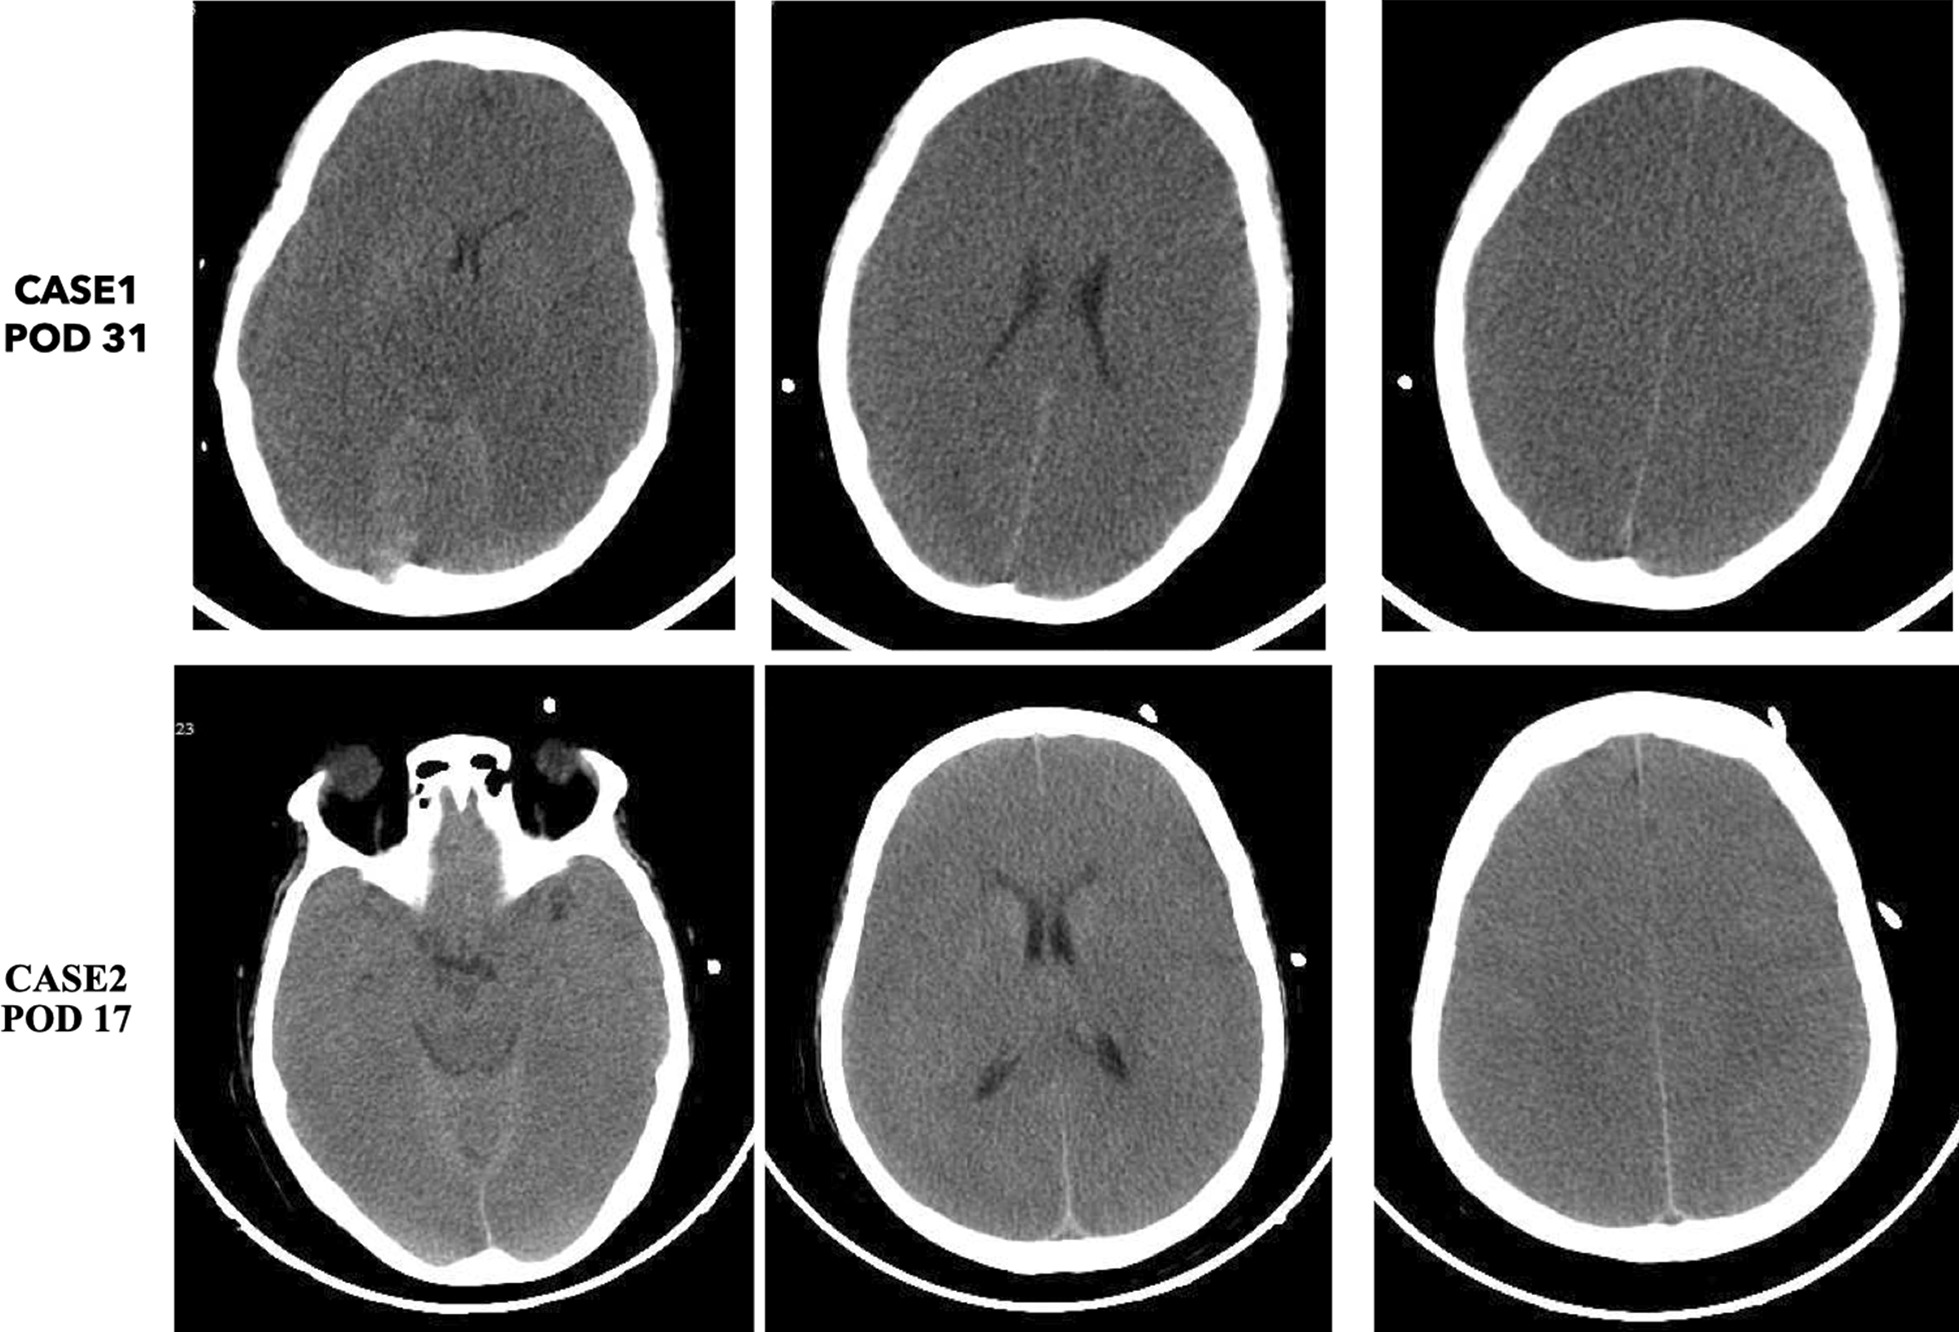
The cranial CT of CASE1 on POD31 (upper panel) and CASE2 on POD17 (lower panel). Cranial CT showed that the density of the brain parenchyma decreased slightly, and some sulci and gyri became shallow and disappeared
